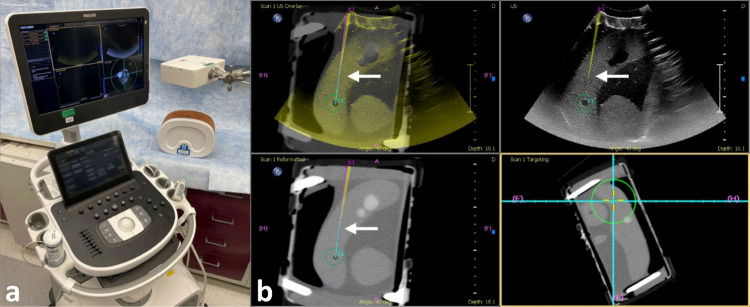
US-based fusion navigation system with EM-tracked needle and US transducer. a Ultrasound-based fusion device consisting of an ultrasound system, electromagnetic field generator and patient tracker. b Tracking display with blended fusion image of ultrasound and CT (top left), orthogonal views that include the tracked needle (top right, bottom left), and a bull’s-eye view along the axis of the tracked needle with the location of the needle centered in the yellow cross and the target centered in the teal lines (bottom right). The purple line is the planned needle path for the paired entry point and target, labeled E1 and T1, respectively. The yellow line is the tracked needle location with the dotted line showing the forward trajectory (yellow dots proximal to the target and teal dots distal to the target). The tip of the needle based on tracked location is indicated by the white arrow. The green circles in the fusion and the two orthogonal views appear if the target is in the image. The radius of the green circle in the bull’s-eye view represents the distance from the needle tip to the plane of the target
